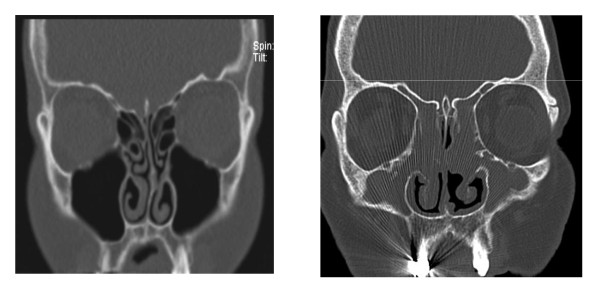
Coronal CT scan images of normal (left) and NP patient (right).
